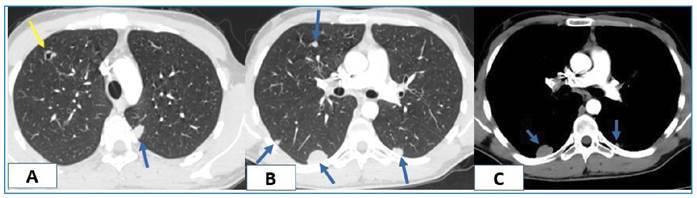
Thoracic CT showing (A) cavitary nodule in anterior segment of the upper lobe of the right lung (yellow arrow) and (B,C) multiple solid-cystic nodules in both lungs (blue arrows).
